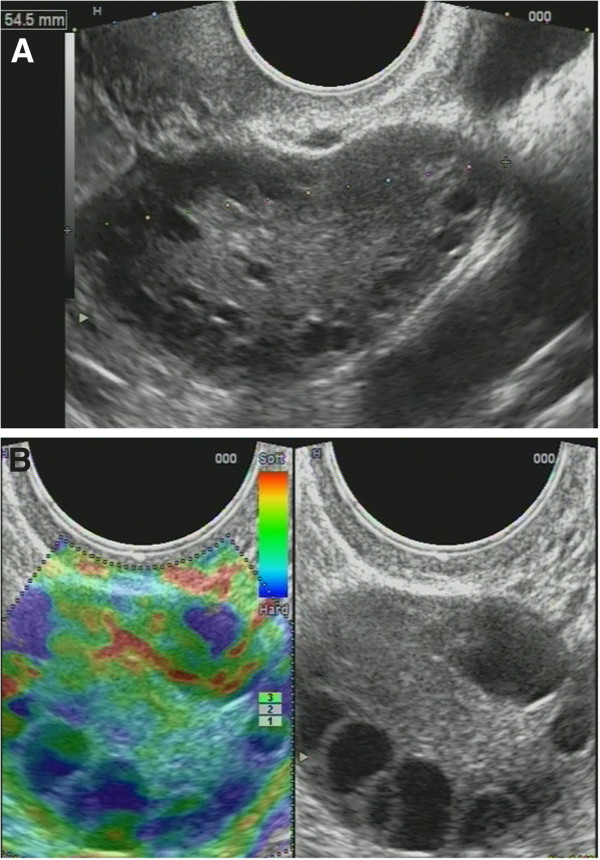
Ultrasonograms of ovaries. (A) Polycystic ovarian syndrome (PCOS) - enlarged to 55 mm in length with small fluid inclusions. (B) Hyperstimulated ovary: BRG artefact in follicles.
